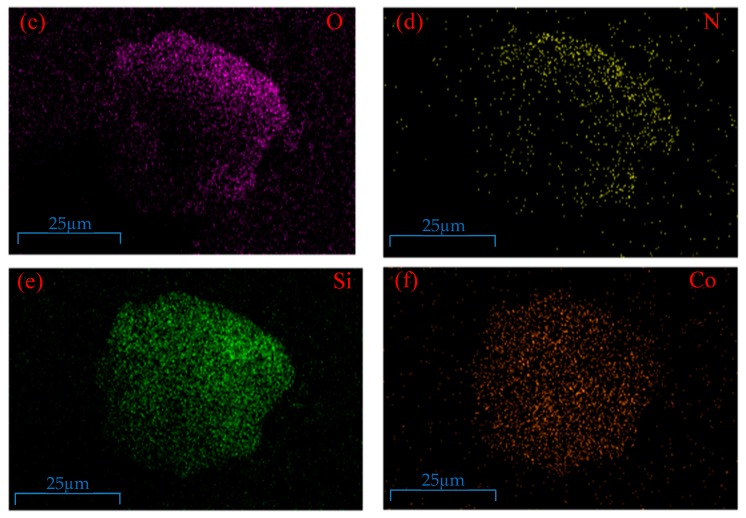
(a) SEM with X-ray area scanning; EDS mappings of (b) Fe, (c) O, (d) N, (e) Si, and (f) Co of CoFe_2_O_4_@SiO_2_-Ppy.
